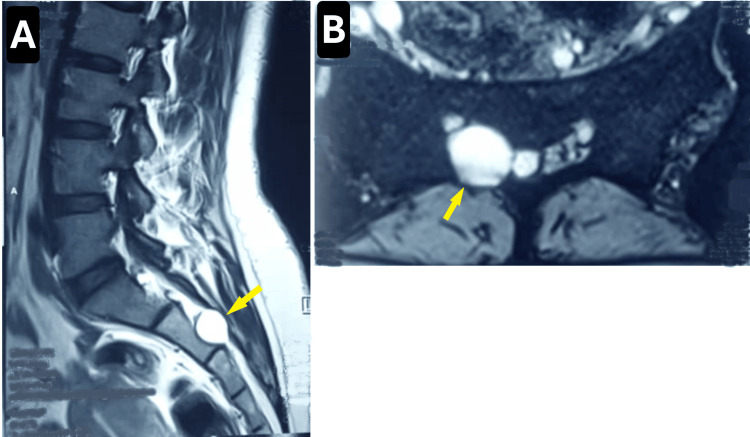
Preoperative MRI findings Sagittal (A) and axial (B) T2-weighted MRI scans show a well-defined, hyperintense, ovoid Tarlov cyst involving the right S2 nerve root, causing compression of adjacent neural structures. Yellow arrows indicate the cyst location.
